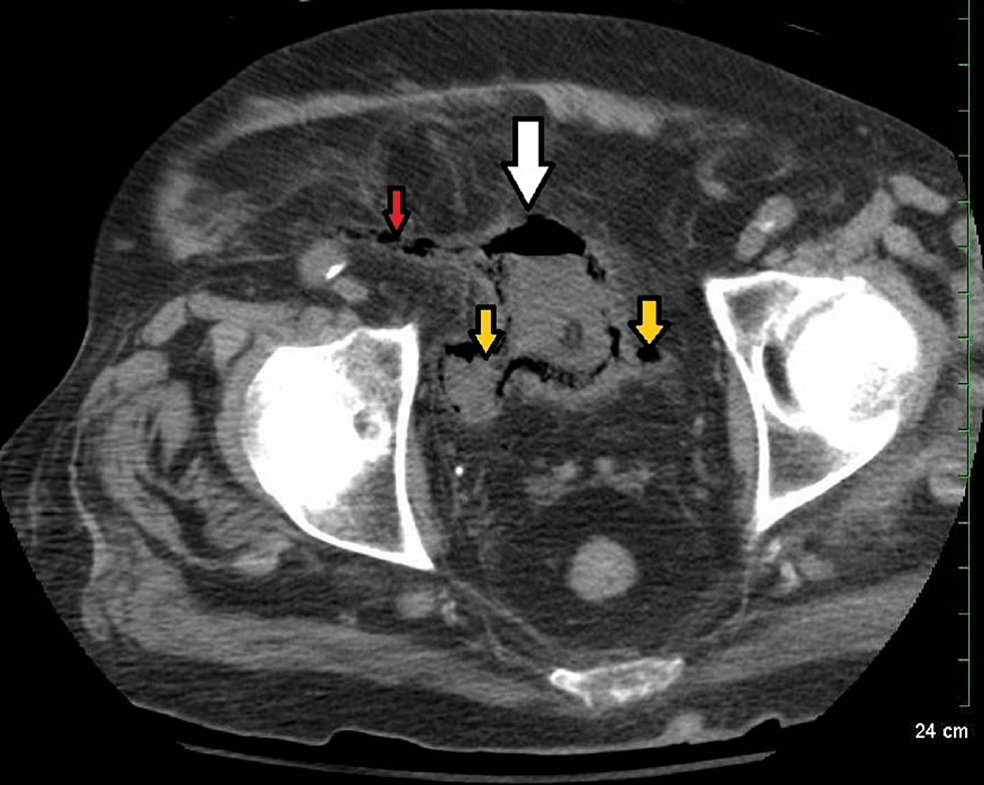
Non-enhanced CT image showing gas in the bladder (white arrow), transplanted distal ureter (red arrow), and native distal ureters (yellow arrow).
